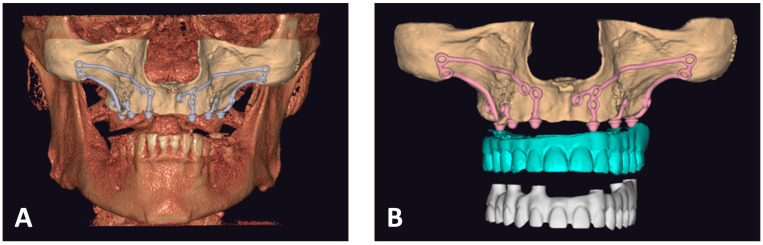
(A) An individual implant after the segmentation process. (B) The Mai Implant^®^ after the superimposition of a CBCT scan image with a removable prosthesis with radiopaque gutta-percha balls.
